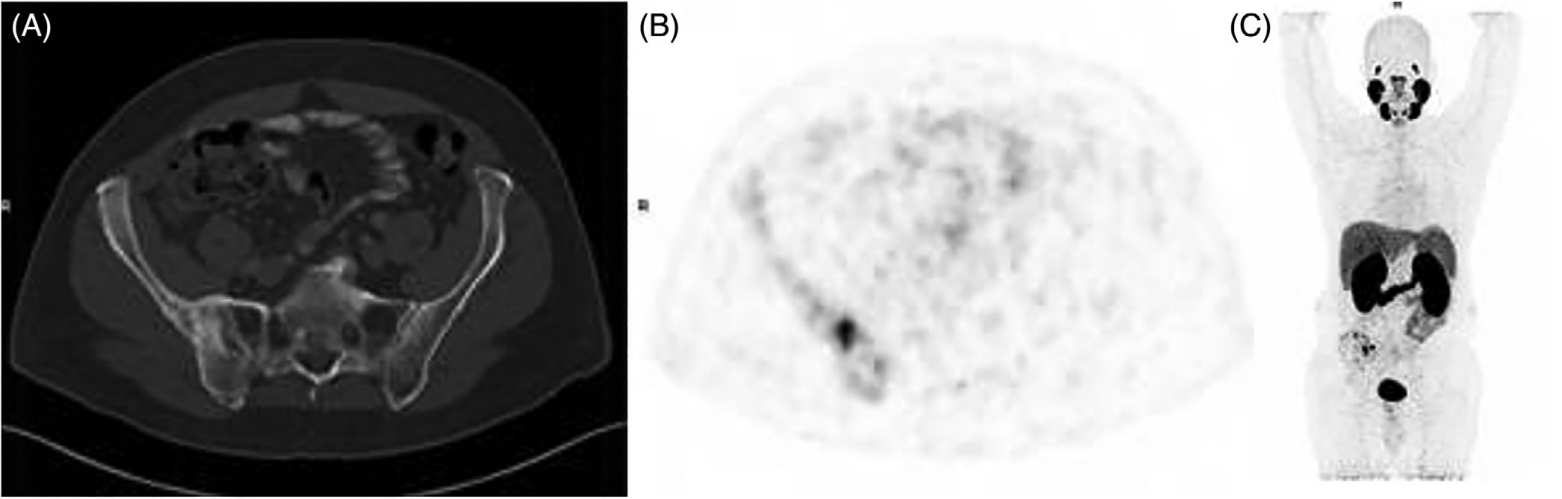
Ga^68^PSMA PET/CT of 65‐year‐old patient with known prostate cancer (Gleason 3 + 4, PSA 4.7) shows sclerotic changes in the right iliac bone A, with highly increased (SUV max 14.6) PSMA accumulation, B and C, MIP maximum intensity projection). Paget disease diagnosed on later performed biopsy
